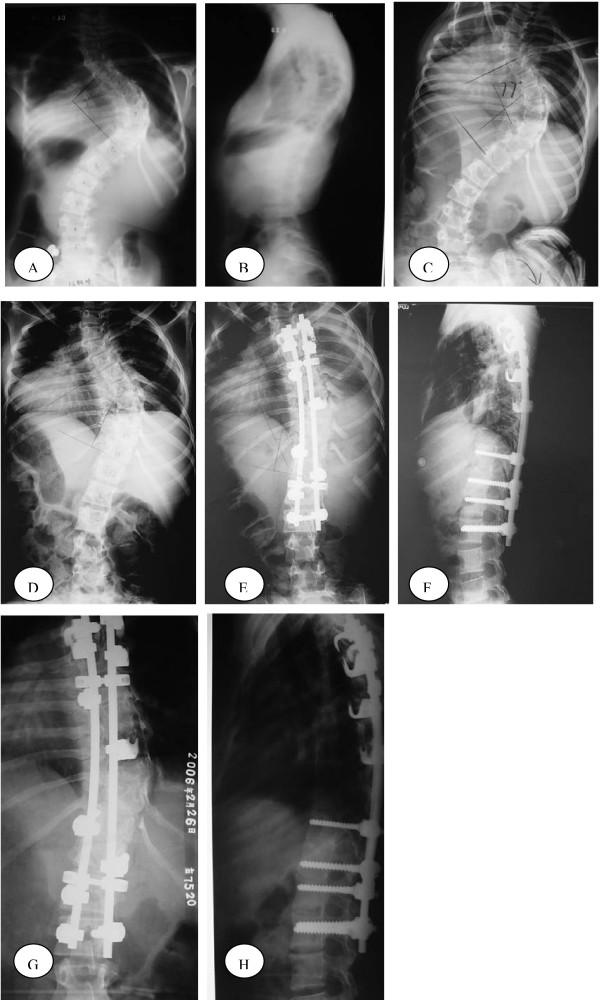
A 14-year-old girl with idiopathic scoliosis and the Lenke classification was 1C+. A-B: AP radiographs before treatment showing right thoracic scoliosis measured 92°. C:Bending films showed right thoracic scoliosis corrected to 77°. D:The right thoracic curve correction obtained with Halo-femoral traction treatment was 40.2%. E-F:The major curve measured 35° after posterior spinal fusion and the correction rate was 62%. G-H: AP and lateral radiographs at 20-month follow-up showed solid spinal fusion with a 37° right thoracic curve.
